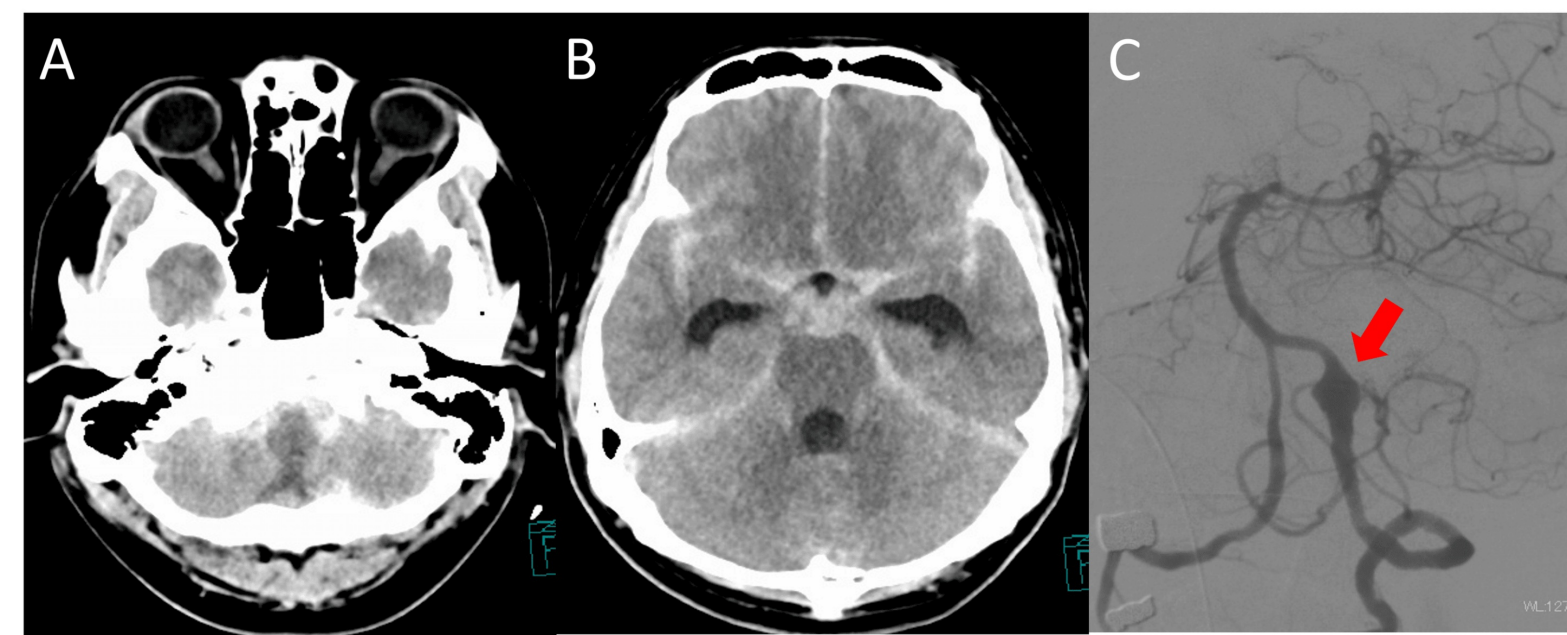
Initial imaging findings. (A) Initial non-contrast head CT demonstrating thick subarachnoid clot around the brainstem. (B) Initial CT showing subarachnoid hemorrhage within the basal cisterns. (C) Initial cerebral angiography demonstrating left vertebral artery dissection (arrow).
